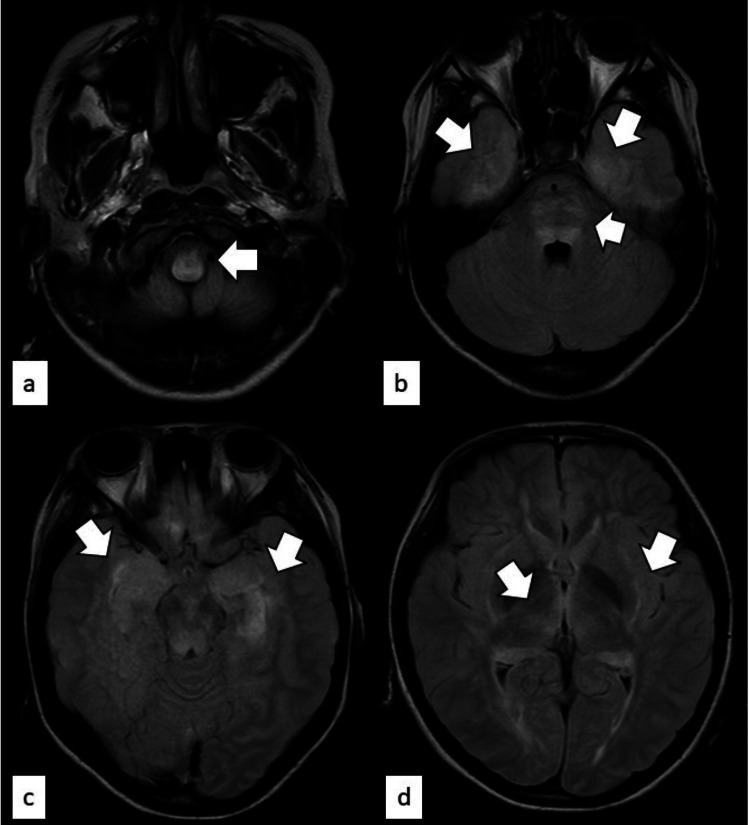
Magnetic resonance imaging scan (fluid attenuated inversion recovery) performed at the time of hospitalization High intensity regions are indicated by arrows as follows: (a) medulla oblongata, (b) medial temporal lobe and bridge capsule, (c) hippocampus, (d) thalamus and external capsule
